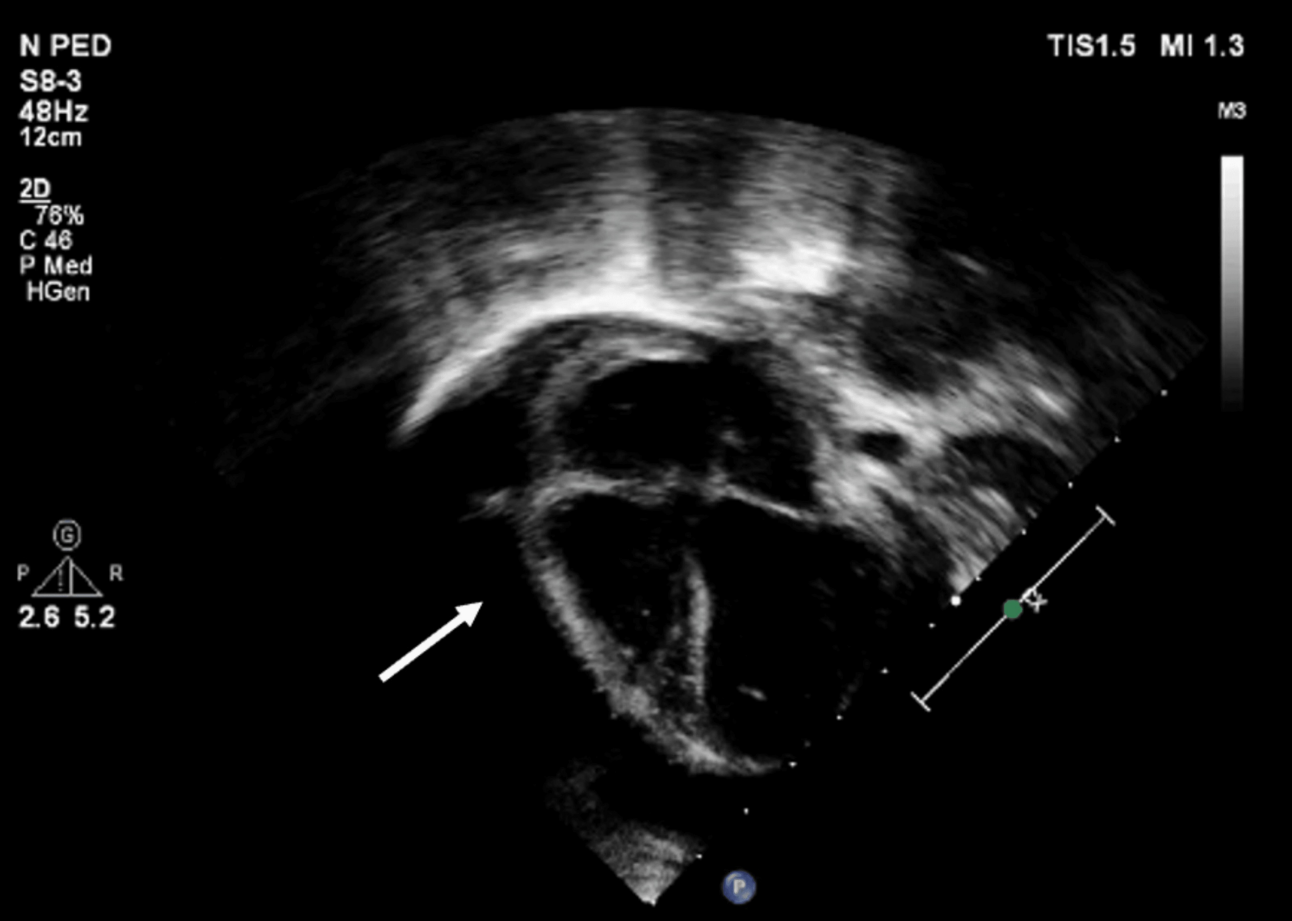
Transthoracic echocardiography (TTE) apical four chamber and parasternal short axis 2D view showing circumferential large pericardial effusion (at the time of presentation)
